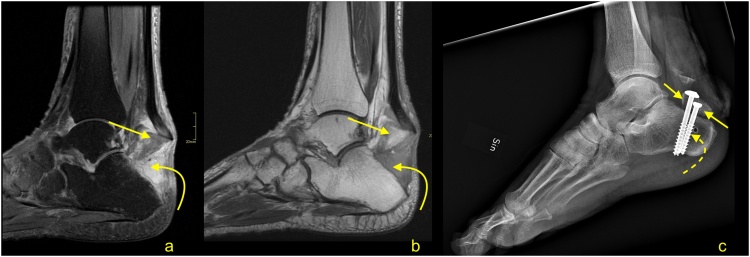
Comminuted avulsion fracture of the calcaneus. Car accident. Large bone fragment (straight arrow) with the attachment of Achilles tendon detached from the calcaneus (a and b- MRI). At the level of the fracture hematoma (curved arrow). X-ray (c) showed that the largest bone fragments are fixed to the calcaneus (dashed curved arrow). The incomplete postoperative function of the Achilles tendon. A- T2- weighted with fat suppression, b- PD- weighted, c- the lateral projection of the ankle joint.
